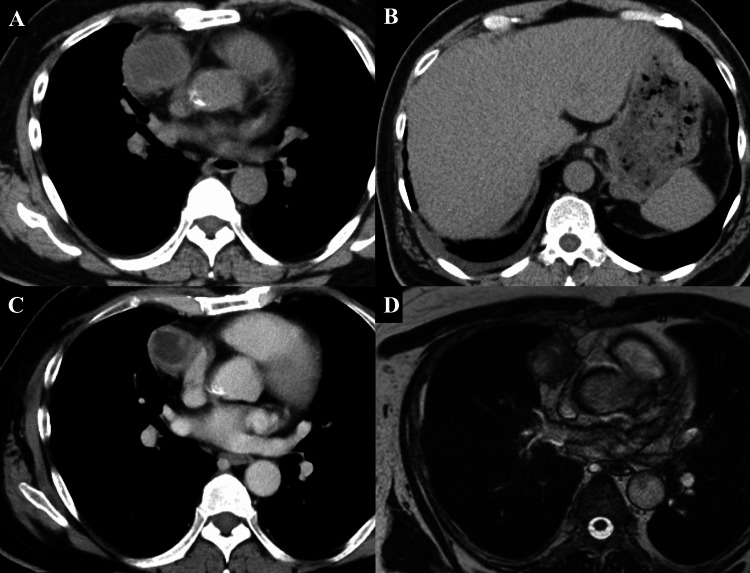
Preoperative radiological findings (A, B) Non-enhanced CT performed at a previous hospital showed a 4.9 cm anterior mediastinal tumor with fat stranding around the tumor and slight right pleural effusion. (C) One month following the preoperative hospital visit, enhanced CT showed that the anterior mediastinal tumor had spontaneously regressed to 3.7 cm. The fat stranding around the tumor and slight right pleural effusion had disappeared. (D) T2-weighted magnetic resonance imaging showed the mediastinal tumor contained a heterogeneous high intensity indicating a cystic change CT: computed tomography
